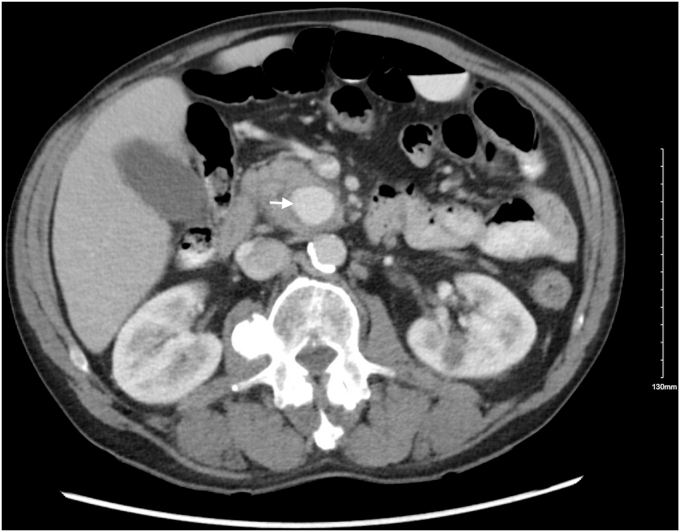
CT of the abdomen showing a pseudoaneurysm measuring 2.6 × 2.4 cm in the uncinate process of the pancreas.
